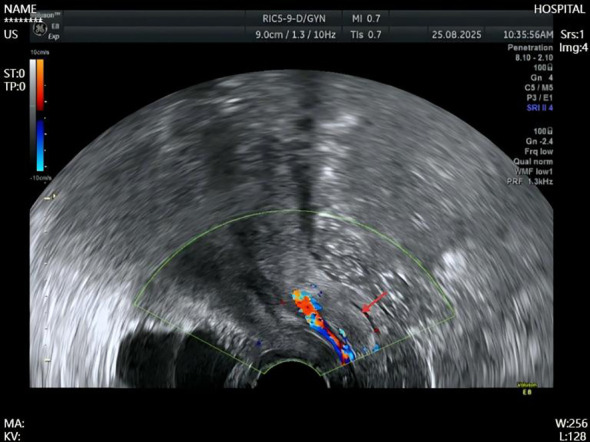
Transvaginal ultrasonographic findings in a 41-year-old patient with vaginal bleeding, demonstrating a 4.2 × 3.1 cm hypoechoic mass within the vagina, with blood flow signals within the lesion and along the posterior wall of the cervical canal. The red arrow indicates the tumor.
